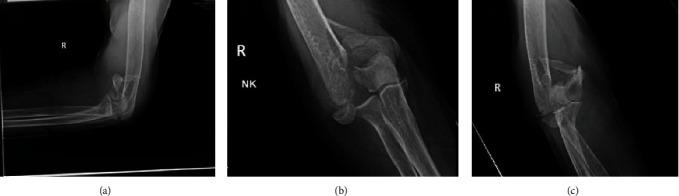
Plain radiograph of the right elbow with (a) the lateral view showing a comminuted distal humeral fracture, (b) AP view showing a bicondylar distal humeral displaced fracture, and (c) showing the right elbow with a two column distal humerus fractures classified as lateral lambda as per the Jupiter classification.
